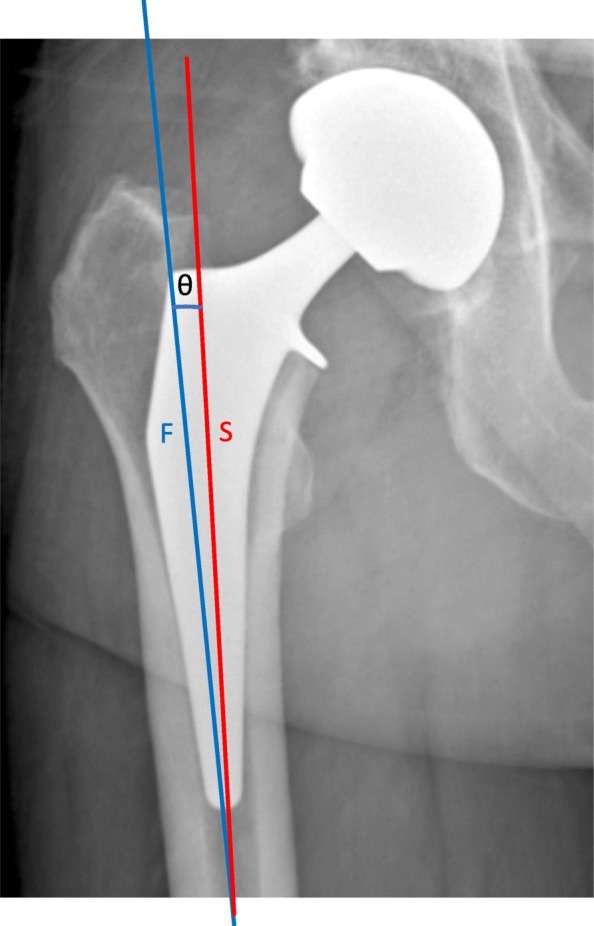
Postoperative radiograph detailing the measurements of stem axial alignment, that is the angle (θ) between the stem axis (S, red; indicated on templates provided by the manufacturer) and the proximal femoral axis (F, blue)
